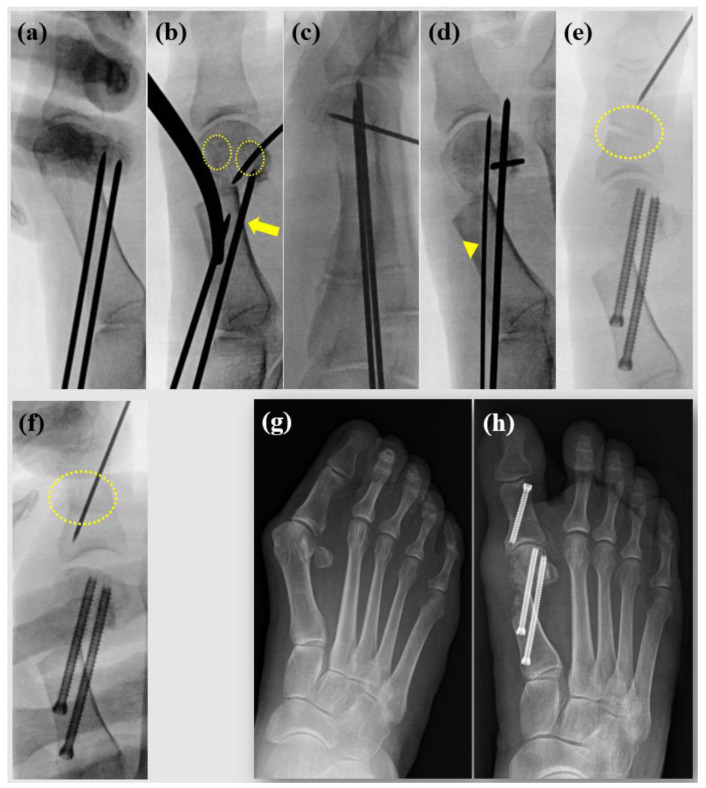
Surgical procedures. (a) Pre K-wiring for ideal screw positioning before osteotomy. (b) Sesamoid reduction with metatarsal head rotation (dotted circles), purchasing lateral cortex of proximal metatarsal (arrow). (c) Check lateral image to confirm proper sagittal alignment. (d) K-wires are exchanged manually to guide wire for screw insertion (arrowhead). (e) During Akin osteotomy procedure, ensuring that the lateral cortex is preserved and is advantageous for precise medial closing, (f) ensure that the medial closing is precisely achieved before inserting the guide pin. (g) Preoperative radiograph. (h) Postoperative radiograph.
